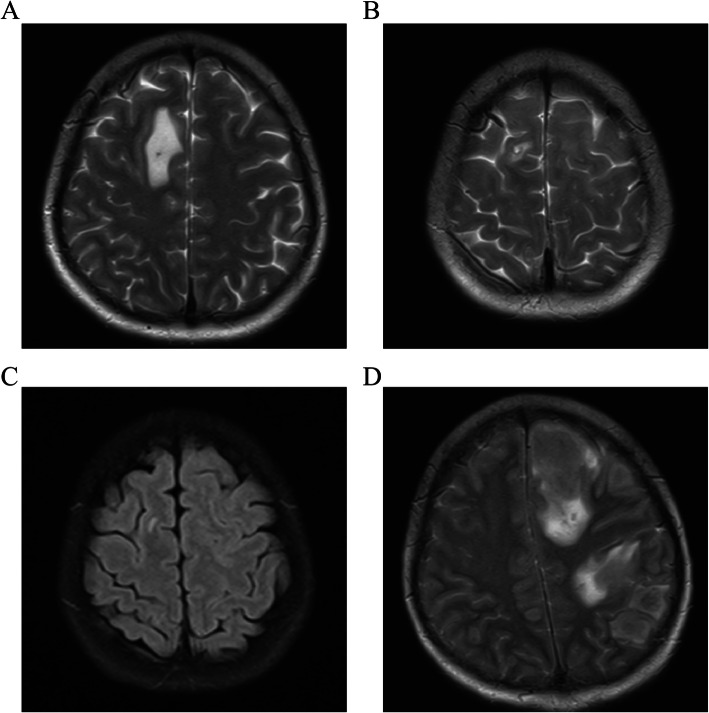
Head MRI for cranial occupancy. A The first cranial MRI. B The second cranial MRI. C The third cranial MRI. D The final cranial MRI
